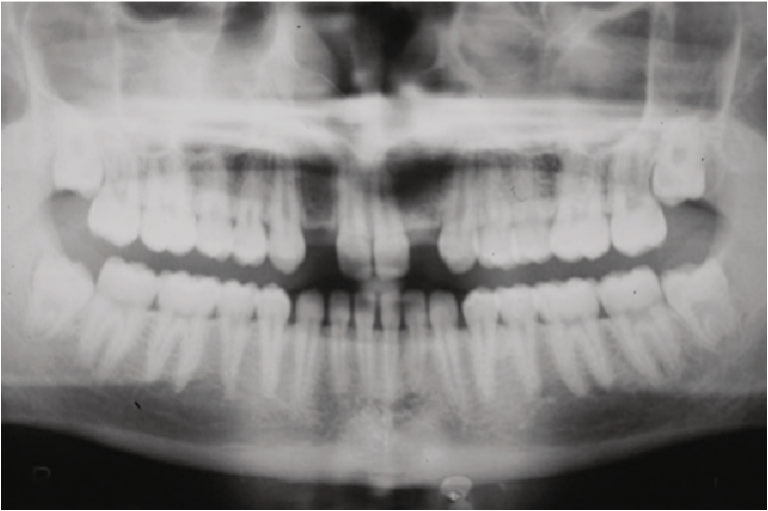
Initial orthopantomography.
